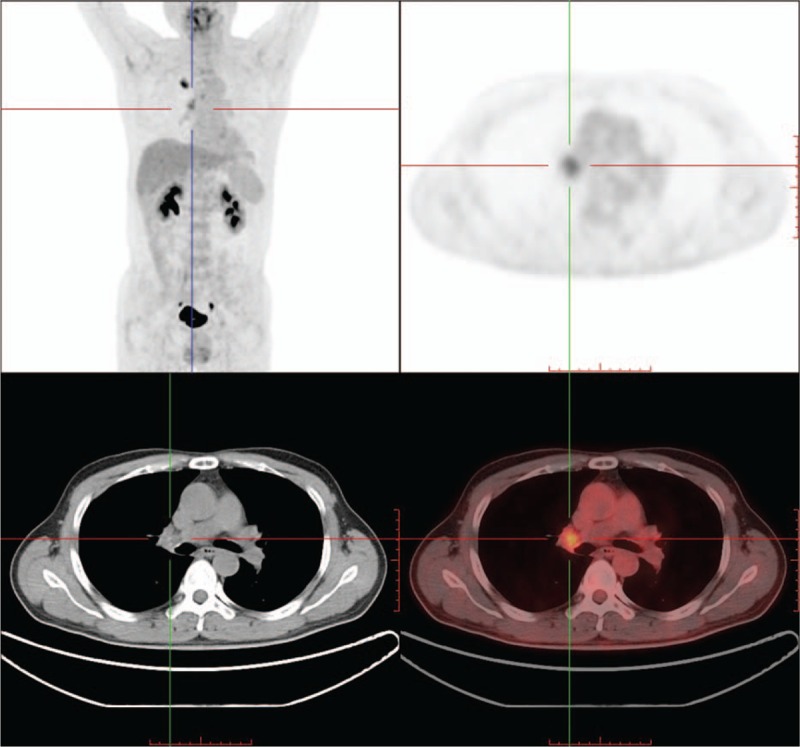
Smoker lung cancer patients positron emission tomography/integrated computed tomography figure. Mediastinal lymph nodes SUVmax value was 2.4 and tumor SUVmax value was 7.9. This positron emission tomography/integrated computed tomography positive mediastinal lymph nodes turns out to be pathological benign.
